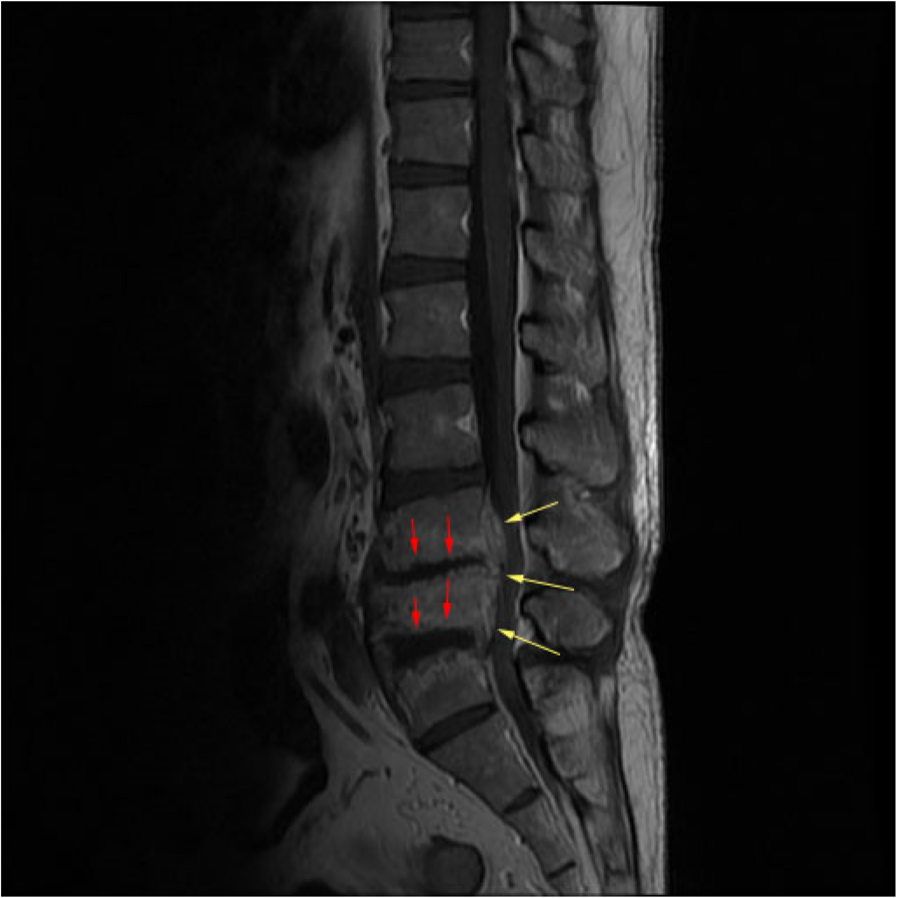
T1-weighted imaging post contrast showing enhancement of the irregular endplate (*red arrows*) with enhancing epidural mass-like lesion (*yellow arrows*)
